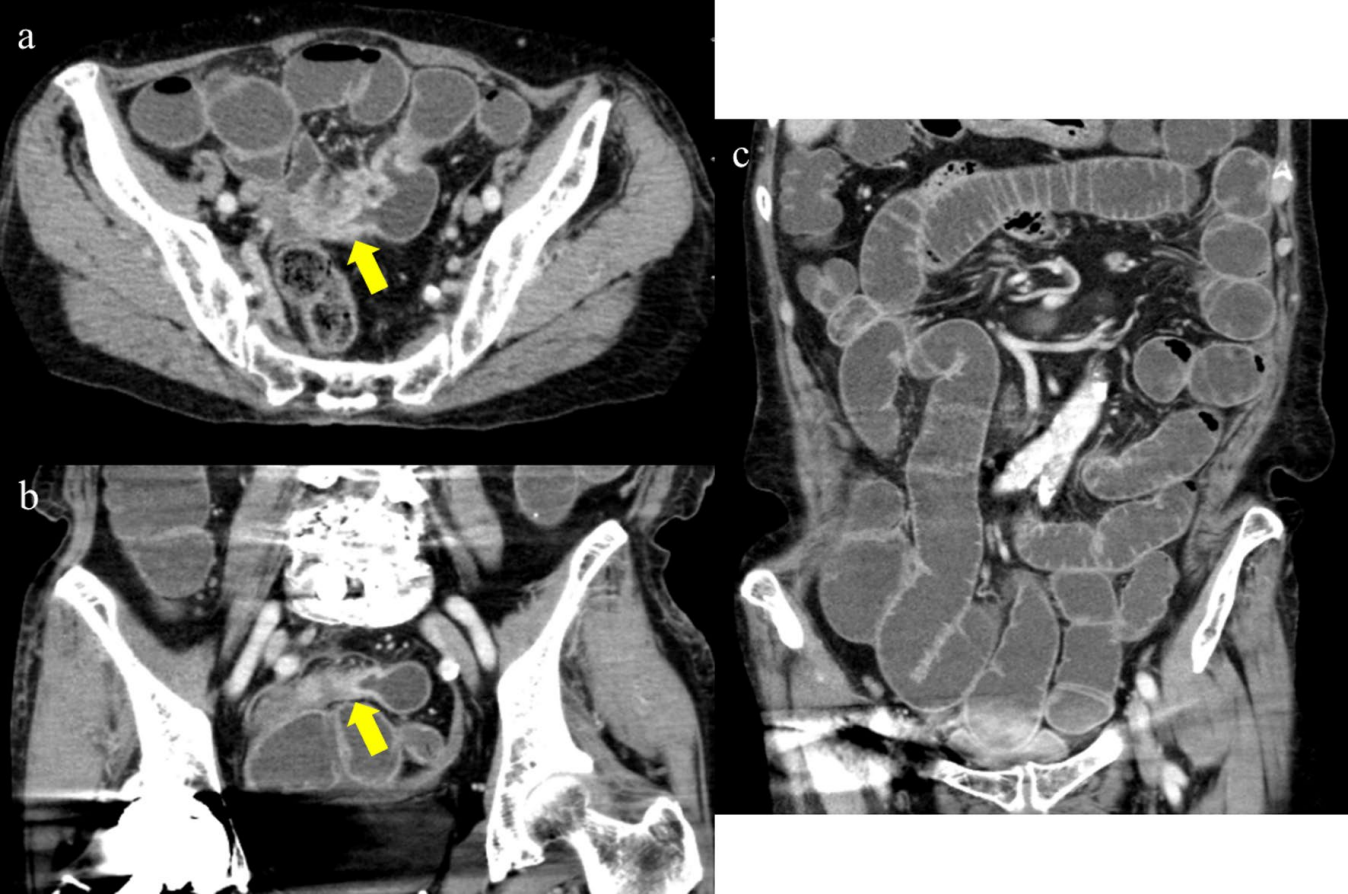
Abdominal CT findings. CT images revealed wall thickening and stricture of the small bowel (arrow) and fat stranding around the lesion (a axial view, b coronal view). A wide range of small bowel distension and fluid accumulation in the lumen were observed, showing small bowel obstruction (c). There were no specific findings indicating disorder of blood circulation or any characteristics, such as calcification over the mesenteric veins and the bowel walls
